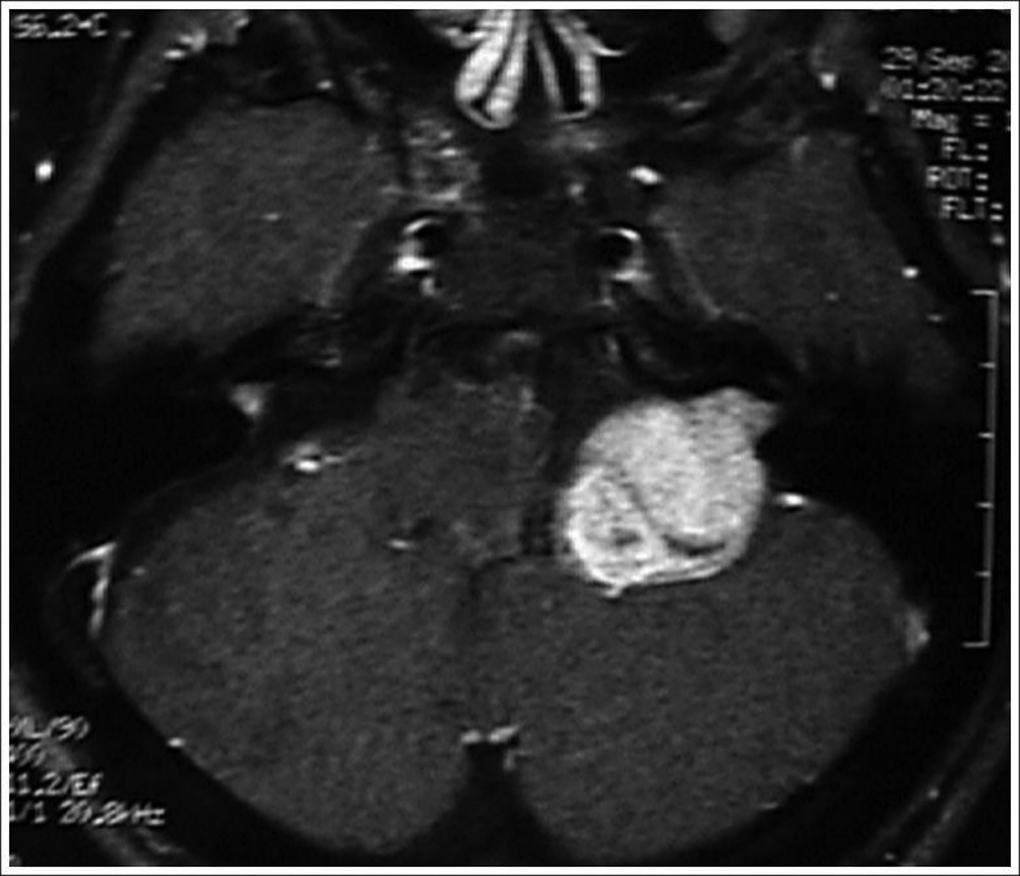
MRI (T1 contrast-enhanced) case 3 preoperative axial view.
